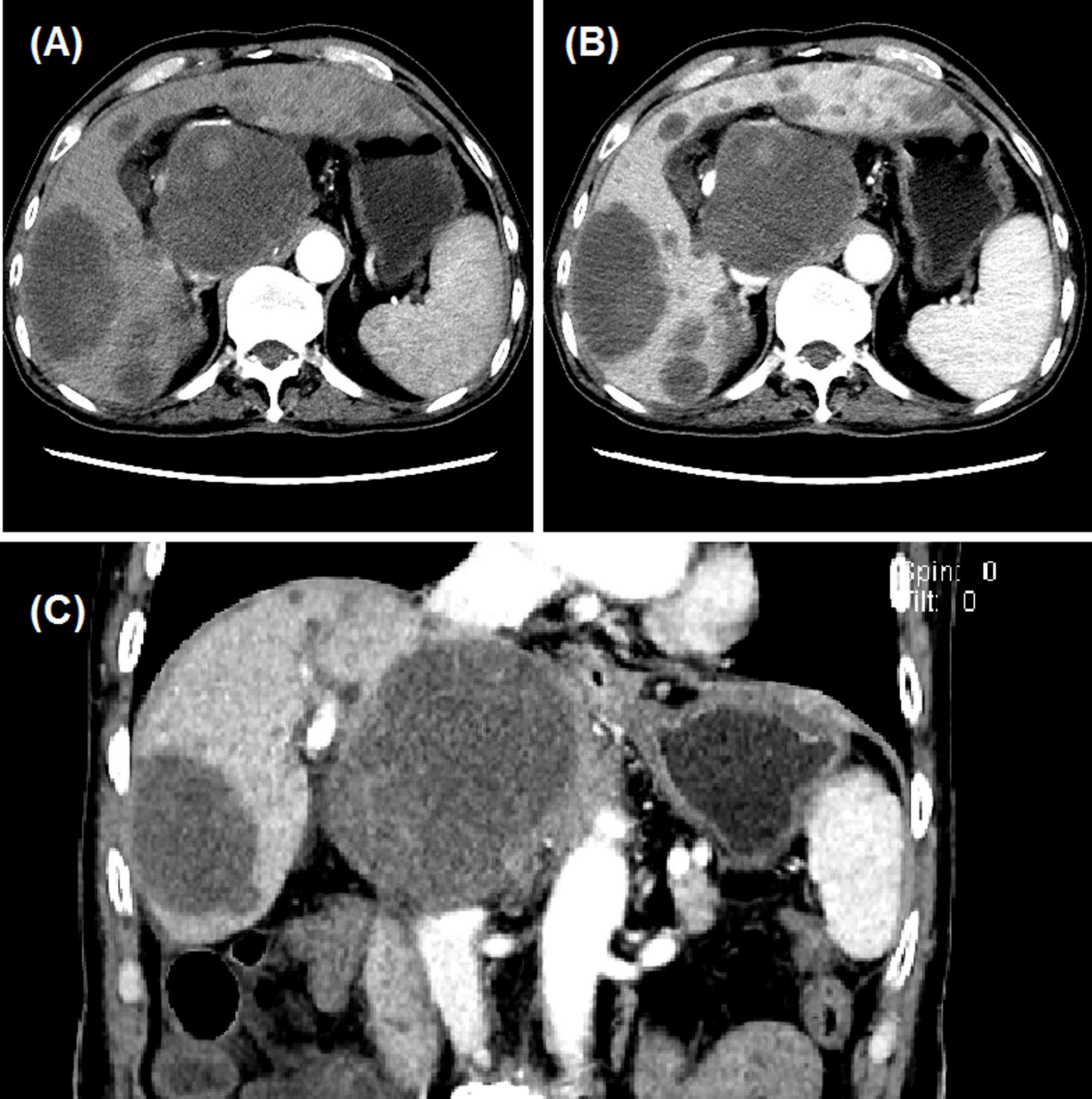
CT imaging follow up for the male patient with advanced primary HCC. (A-C) After 1.5 months’ follow-up, the tumour lesions were found to shrink, with almost no enhancement. PR was assessed according to mRECIST.
